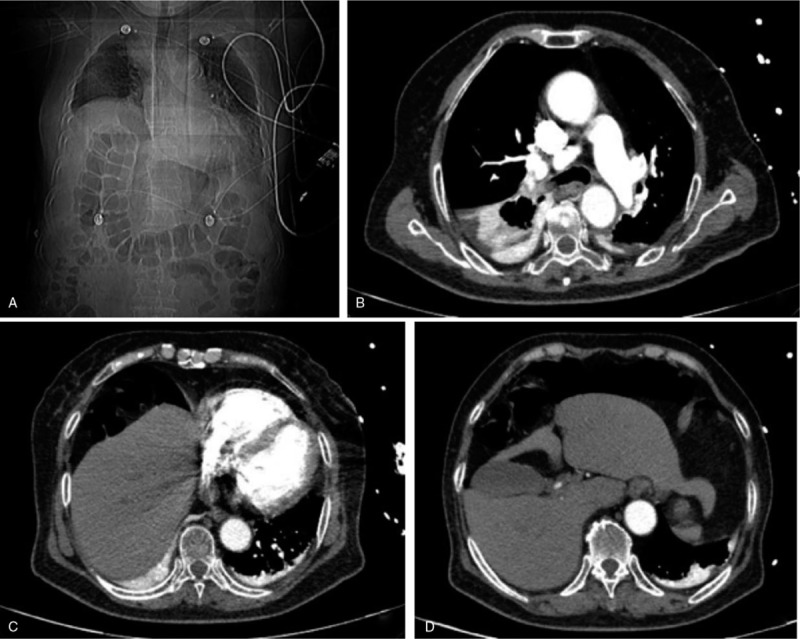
CT showing no signs of pulmonary embolism, leg vein thrombosis, or patchy lung consolidation (A and B). There is upward displacement of the diaphragm along with left-sided shift of the mediastinum (C). The colon is seen located between the liver and the diaphragm (D).
